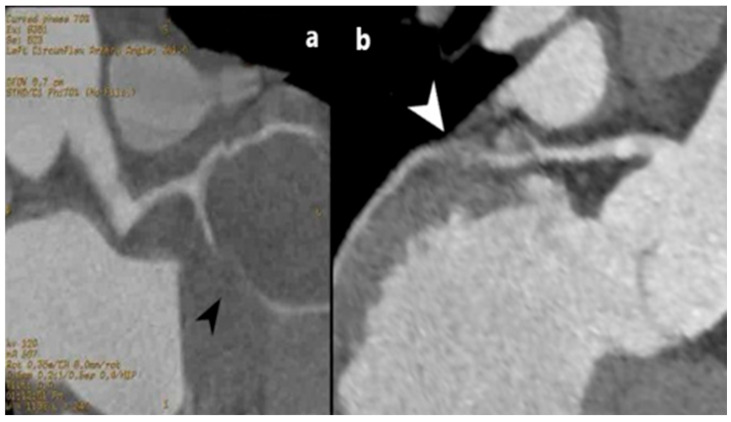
MD CT coronary angiography showing (a) spontaneous coronary artery dissection (black arrowhead); (b) plane image presenting the separation of the inner layer of a coronary artery wall, with formation of an intramural hematoma (white arrowhead). The presented patient was a postpartum woman that presented with symptoms of severe, radiating chest pain. She was initially referred for evaluation due to a clinical suspicion of pulmonary thromboembolism. She was managed conservatively using optimal medical therapy, which included dual antiplatelet agents and beta-blockers, in accordance with current clinical guidelines.
